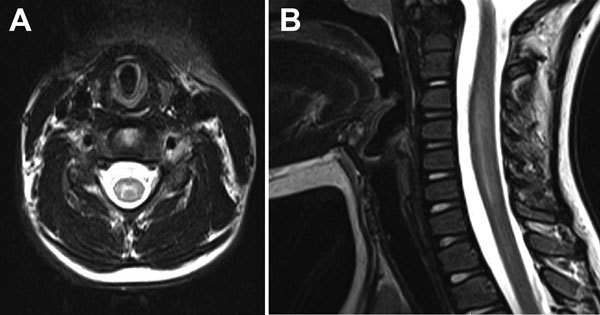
Magnetic resonance imaging of 6-year-old girl with flaccid paralysis and enterovirus C106 infection, Virginia, USA, October 2014. A) Axial T2-weighted image of the cervical spine demonstrating abnormal hyperintensity of the central gray matter (right to left). B) Sagittal T2-weighted image of the cervical spinal cord demonstrating faint longitudinally extensive central hyperintensity and associated cord edema.
